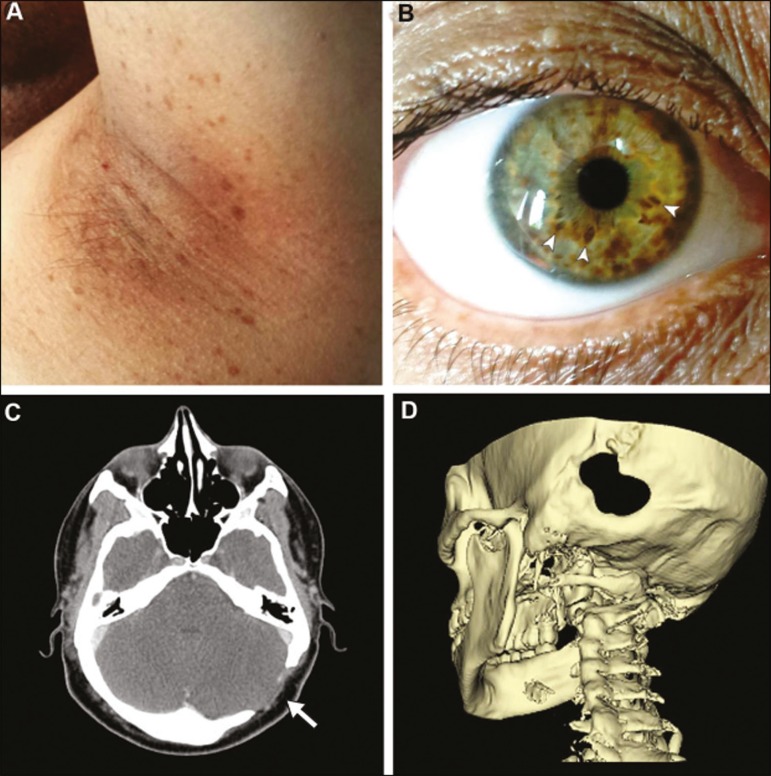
Clinical and CT findings. Bilateral axillary freckles (A) and iris
